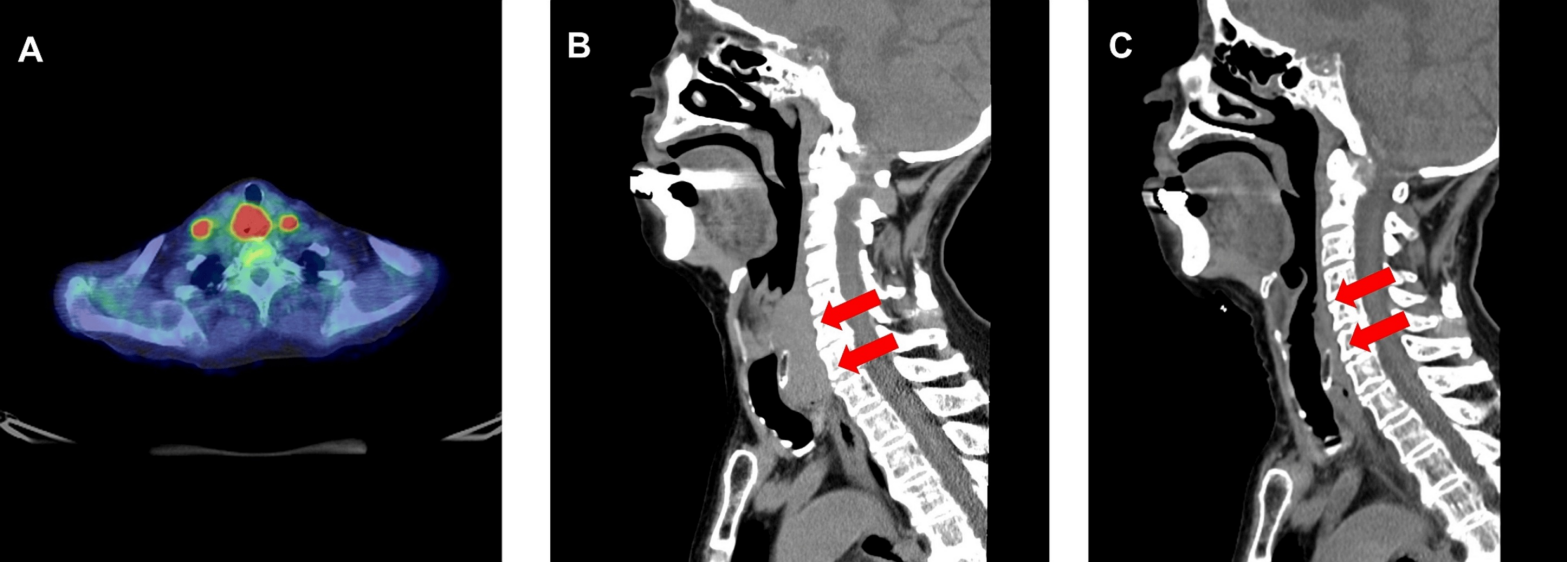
Radiological imaging of lymphoepithelial carcinoma of the hypopharynx in a nonagenarian (A) Pretreatment positron emission tomography-computed tomography (PET/CT) image showing intense fluorodeoxyglucose uptake in the red area of the primary tumor and the involved cervical lymph nodes. (B) Pretreatment sagittal CT scan revealing a hypopharyngeal mass in the cervical region prior to radiotherapy, as indicated by red arrows. (C) A post-treatment sagittal CT scan was performed three months after radiotherapy and demonstrated a marked reduction in the lesion size, indicated by red arrows.
